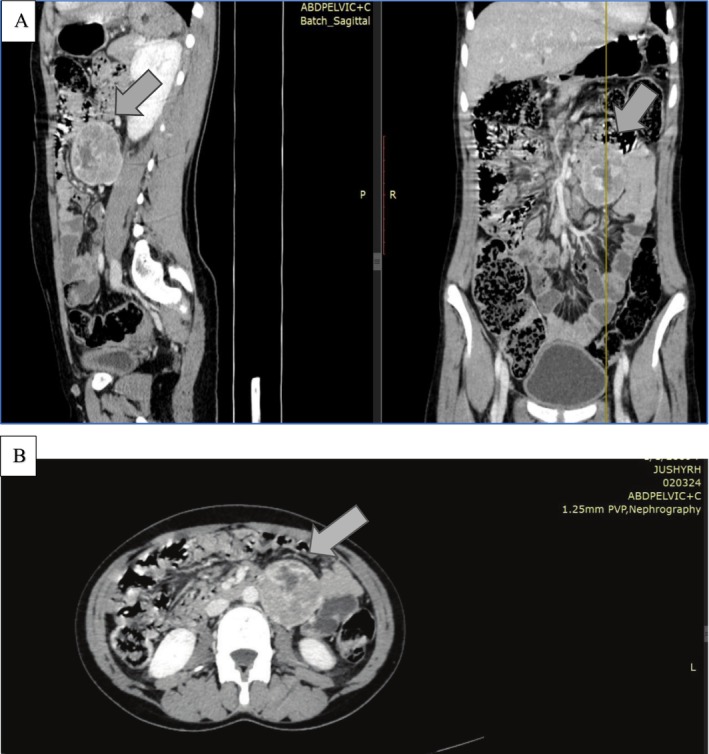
(A) Contrast‐enhanced sagittal and coronal CT views showing a left para‐aortic mass with heterogeneous enhancement and central necrosis. (B) Contrast‐enhanced axial CT view showing the 5.4 × 4.8 × 4.4 cm left para‐aortic mass suggestive of an extra‐adrenal paraganglioma (Organ of Zuckerkandl).
